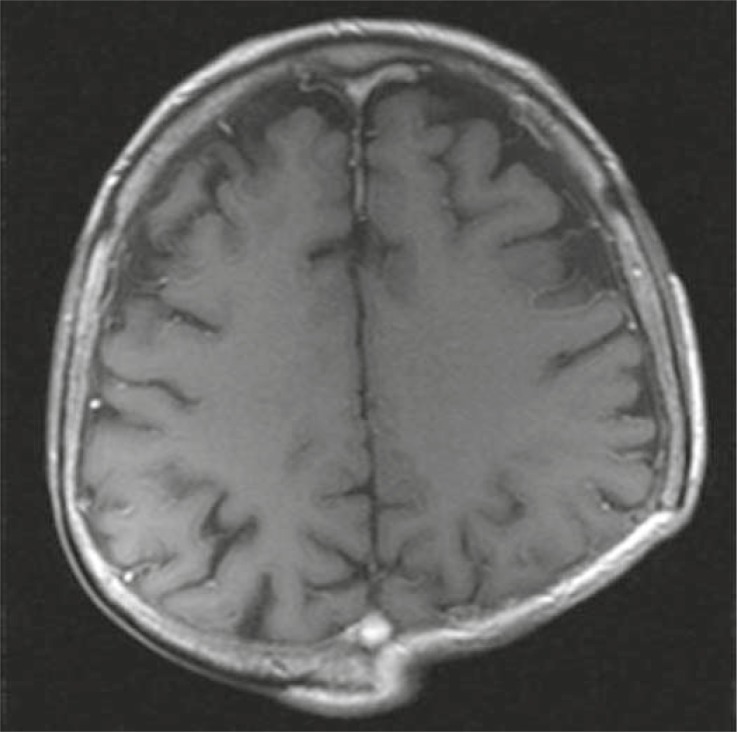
MRI of the brain 9-month later of the XRT.
